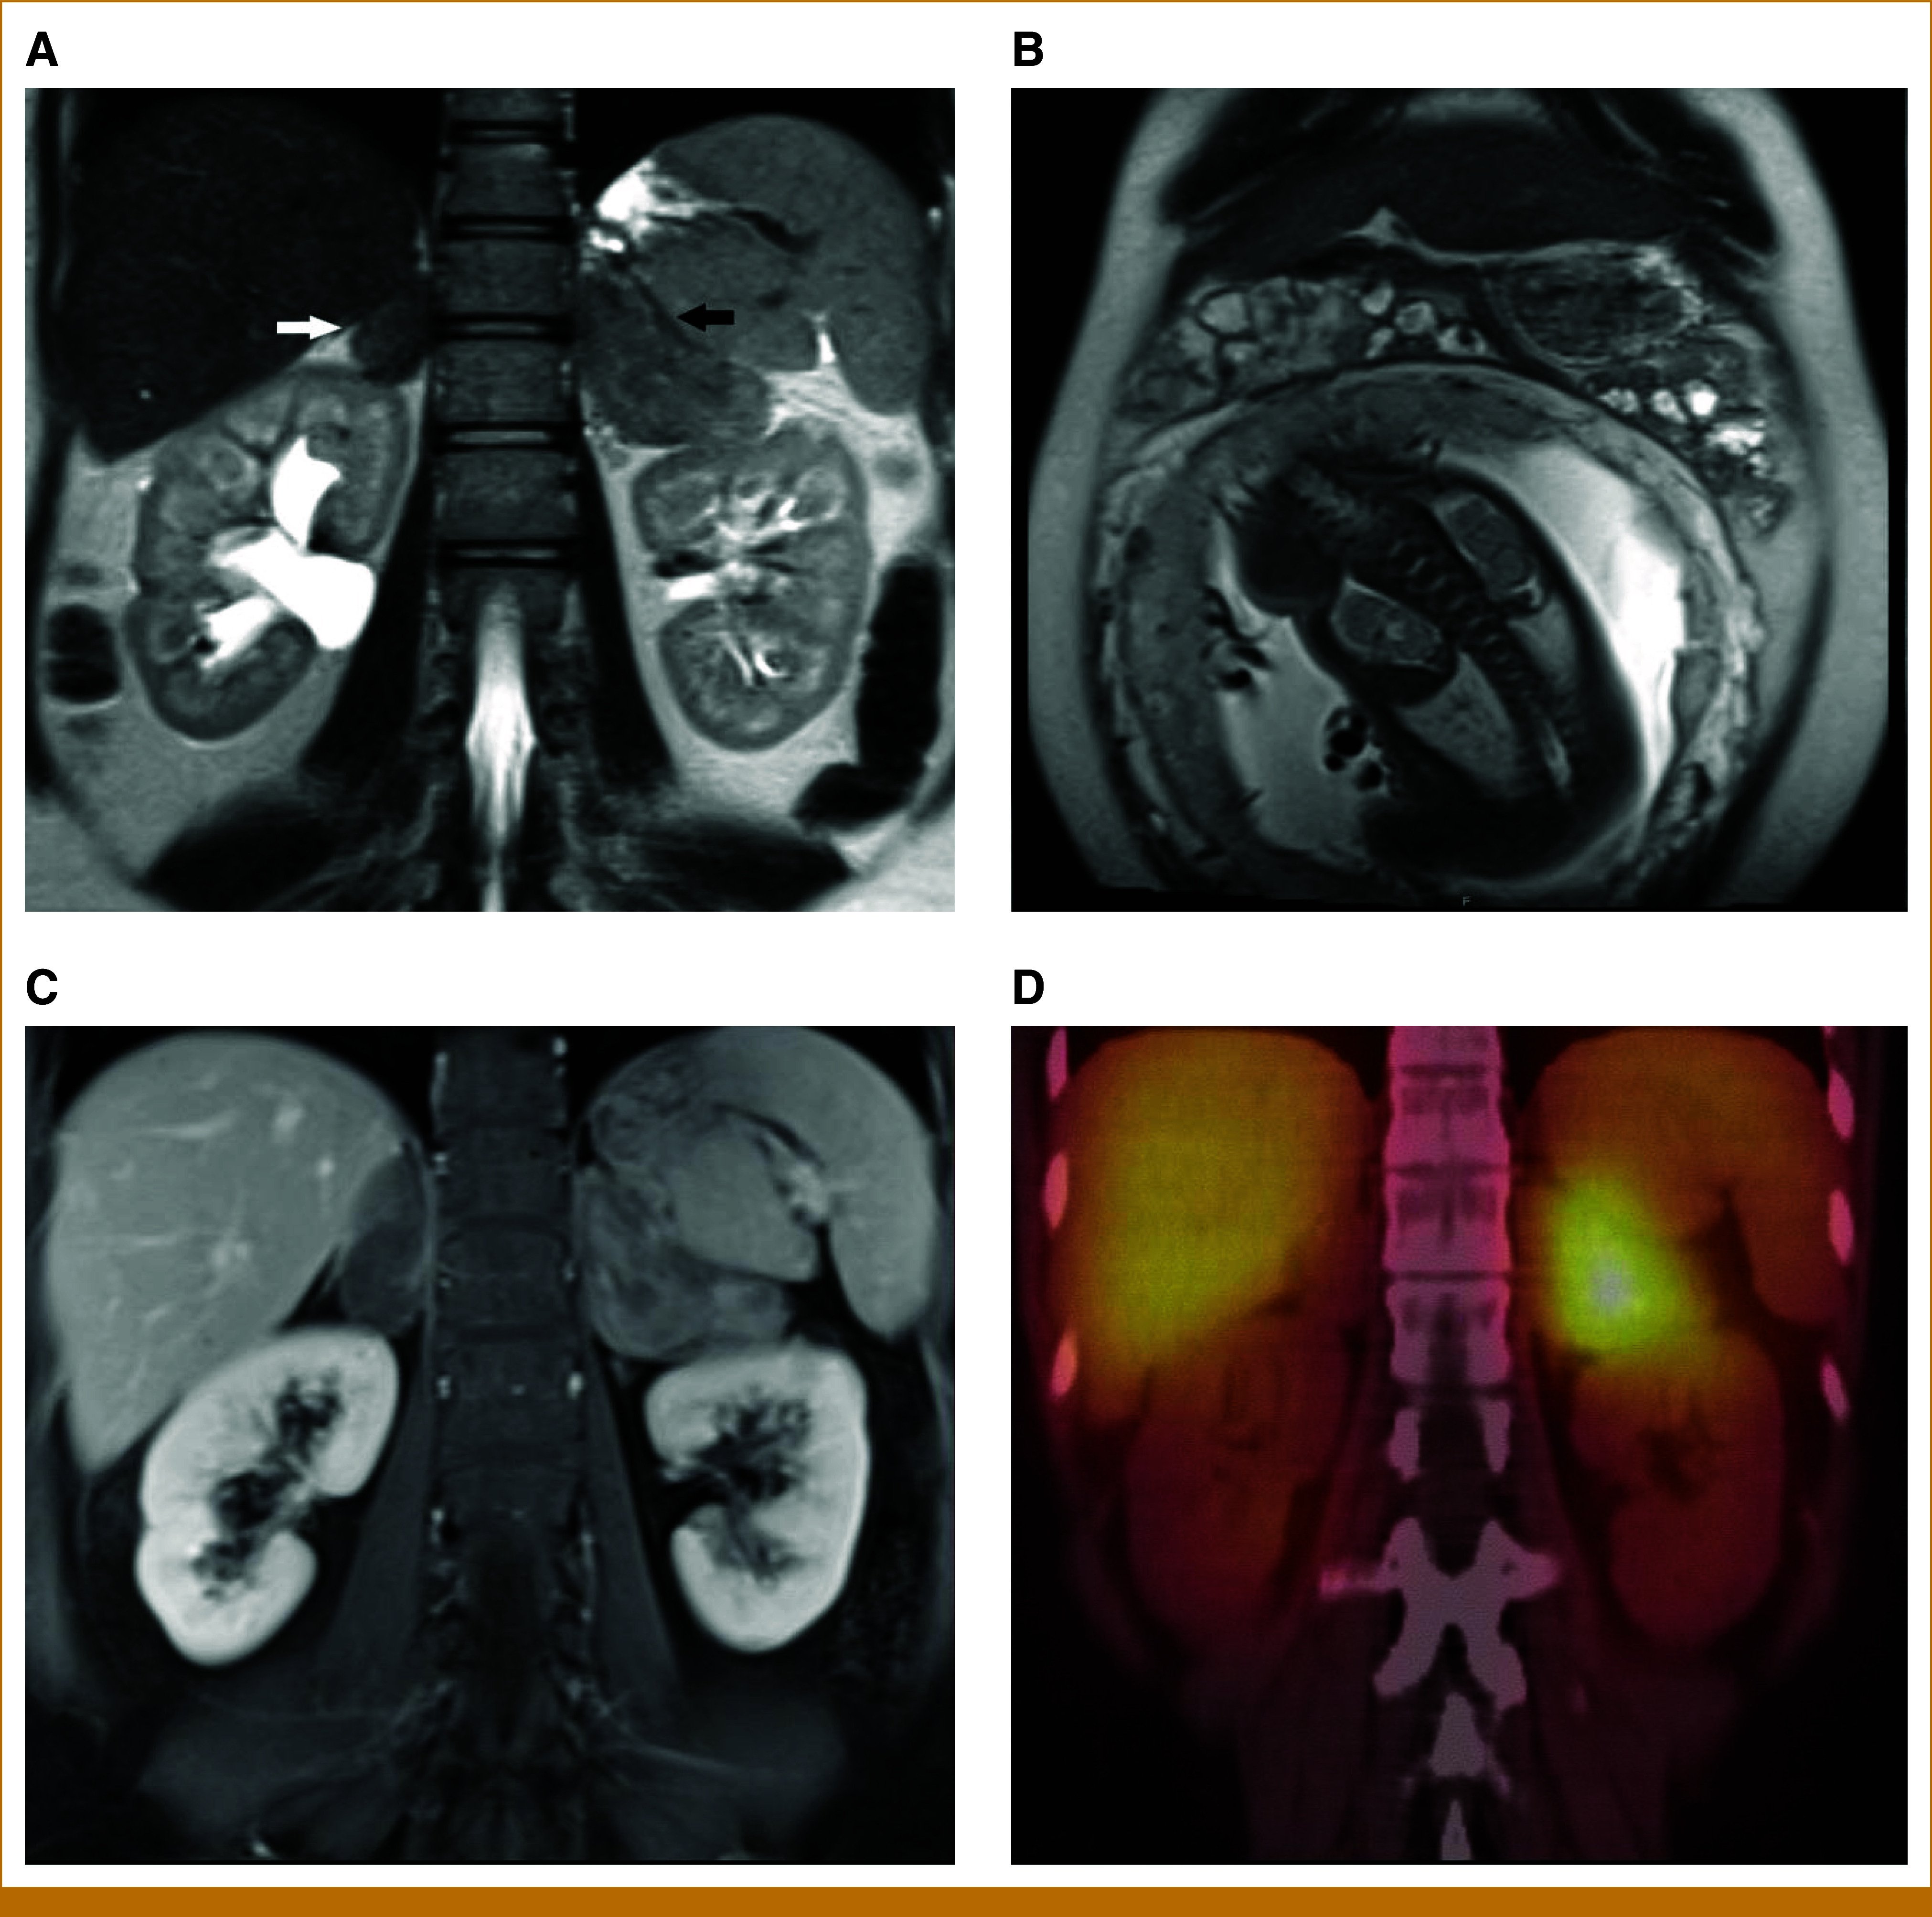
Radiographic findings. (A) Coronal T2-weighted image shows a small, ovoid right adrenal mass (white arrow) and larger, triangular left adrenal mass (black arrow) with heterogeneity and higher T2 signal intensity than the right adrenal mass. (B) Coronal T2 HASTE fetal MRI image shows a fetus with normal adrenal glands. (C) Two months later, postpartum, a contrast-enhanced coronal T1-weighted image with fat saturation shows asymmetric enhancement, mild and homogeneous on right and moderate and heterogenous on left. (D) Coronal I-123MIBG SPECT/CT-fused image shows no uptake in right adrenal mass and moderate uptake in left adrenal mass. MIBG, metaiodobenzylguanidine; SPECT/CT, single-photon emission computed tomography/computed tomography.
